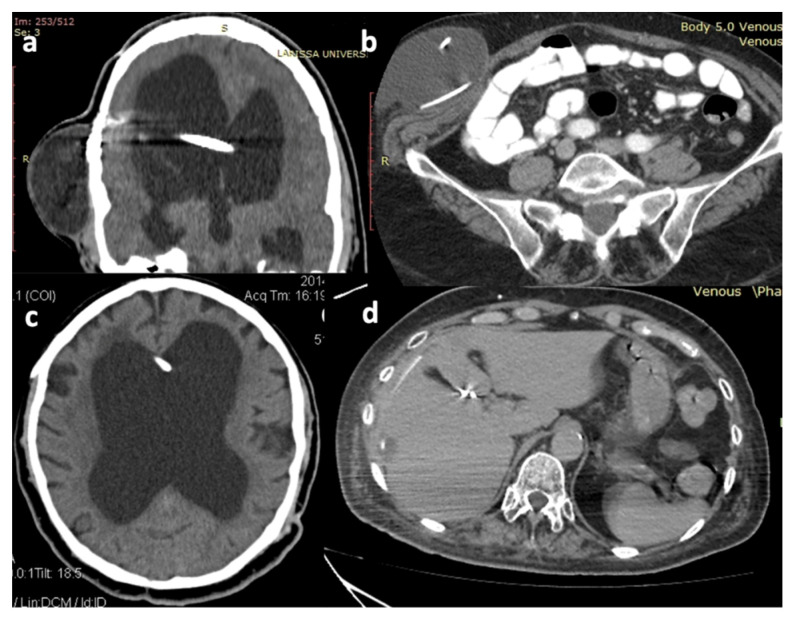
Head and abdominal CT scans demonstrating characteristic cases of VP shunt malfunctions: (a). coronal head CT image showing obstruction of the ventricular catheter leading to subcutaneous CSF infusion, (b). axial abdominal CT image showing subcutaneous CSF infusion due to migration of the inserted peritoneal catheter into the abdominal wall, (c). axial head CT image showing retraction and obstruction of the previously inserted ventricular catheter with enlargement of the ventricular system, and (d). axial abdominal CT image showing peripheral migration of the peritoneal catheter into the liver parenchyma.
